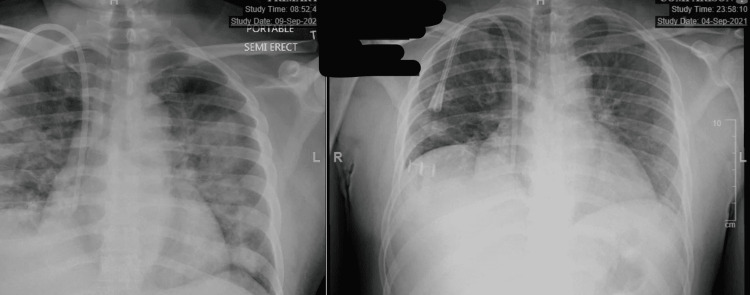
On the left is a chest X-ray done on day four of admission showing a worsening of the lungs. And on the right is the chest X-ray on the day of admission.
